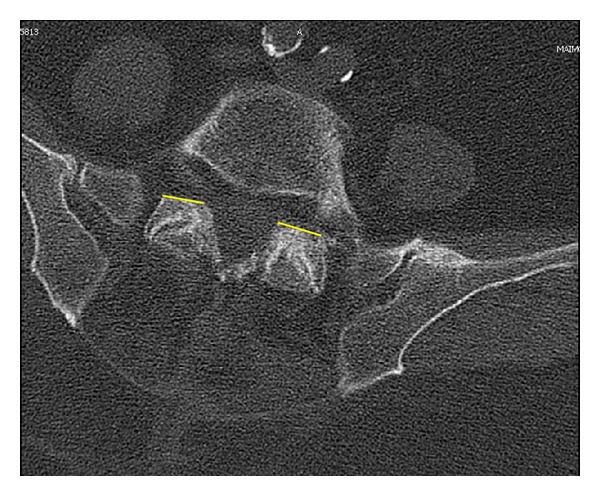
Postoperative axial CT scan image at the level of the facet joint demonstrating partial removal of the upgoing facets.
